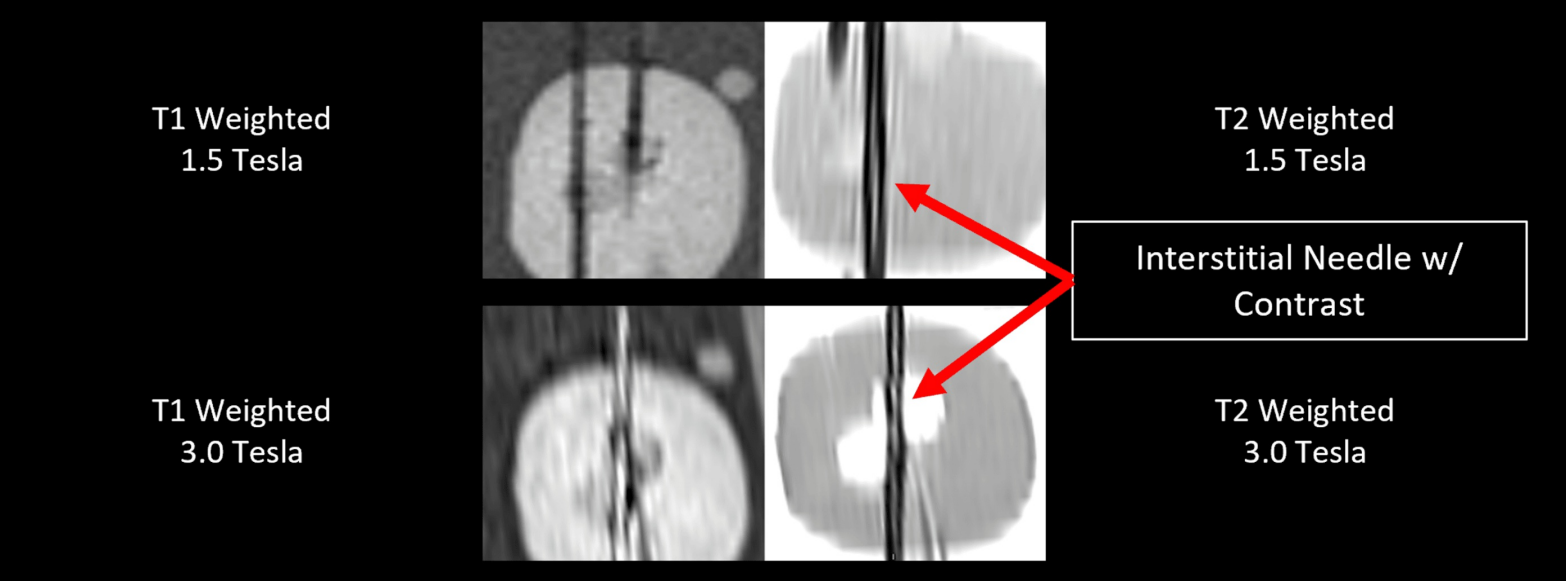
Sagittal slices of MR images taken at different B0-field strengths and pulse sequences. Top left: 1.5 T, T1-weighted; top right: 1.5 T, T2-weighted; bottom left: 3.0 T, T1-weighted; bottom right: 3.0 T, T2-weighted. Markers were visible on all scans except for 1.5 T, T1-weighted.
